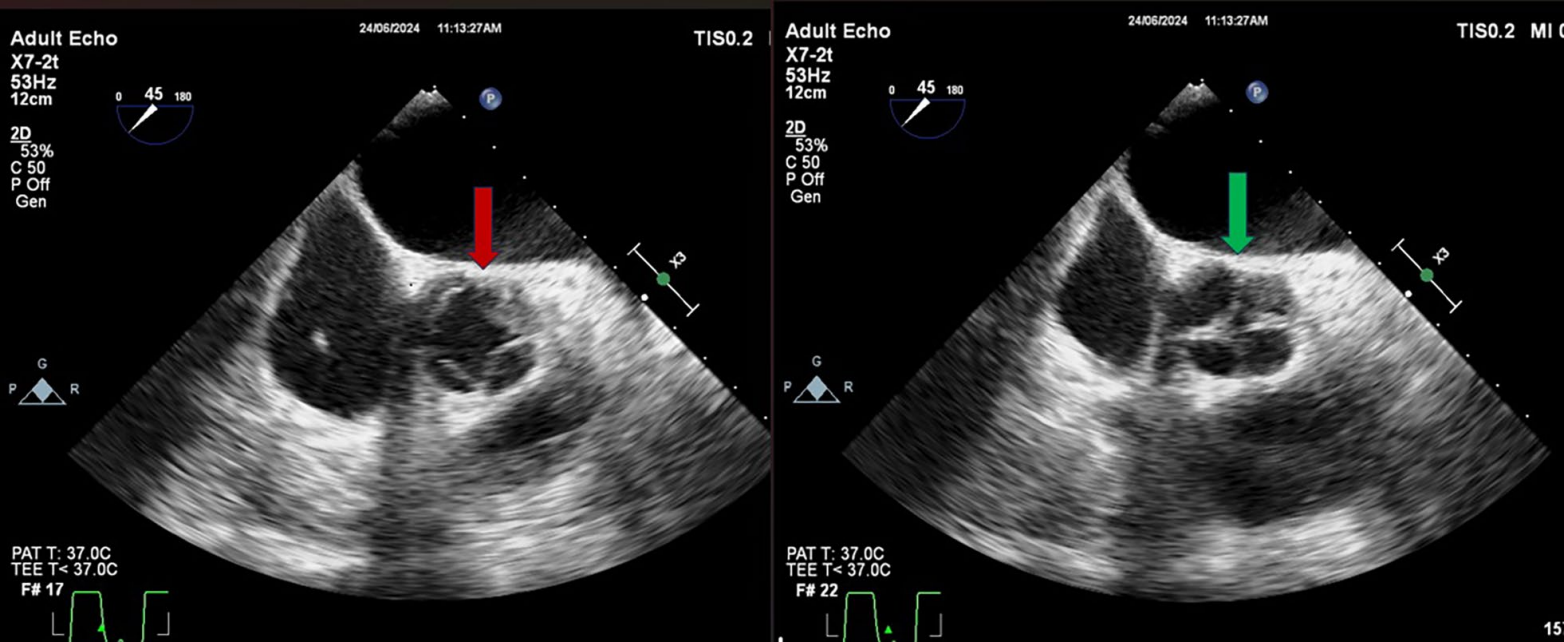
Transesophageal echocardiogram (TEE) image showing aortic valve with 4 cusps in systole (solid red arrow) and diastole (solid green arrow). Note the configuration of “X” in diastole
